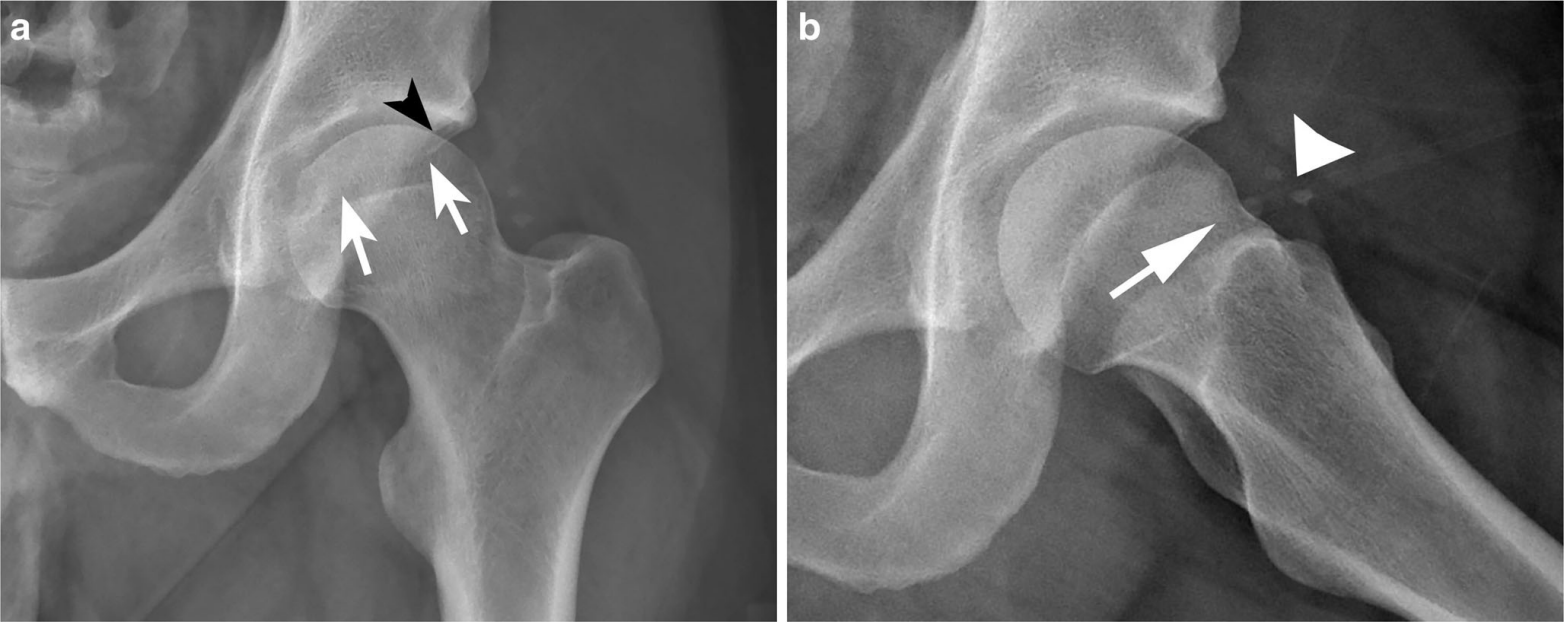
A 22-year-old man with persistent pain after surgery, and imaging suggesting inadequate resection of impingement lesions. a Anteroposterior (AP) radiograph shows “crossover sign” indicating persistent retroversion of the superior acetabular rim.* White arrows* show the anterior acetabular rim crossing over the posterior rim (*black arrowhead*). b Frog leg lateral radiograph shows a shallow indentation at the femoral head–neck junction representing the surgical resection (*arrow*). The head–neck junction remains prominent. There is also a small amount of heterotopic ossification (*arrowhead*)
